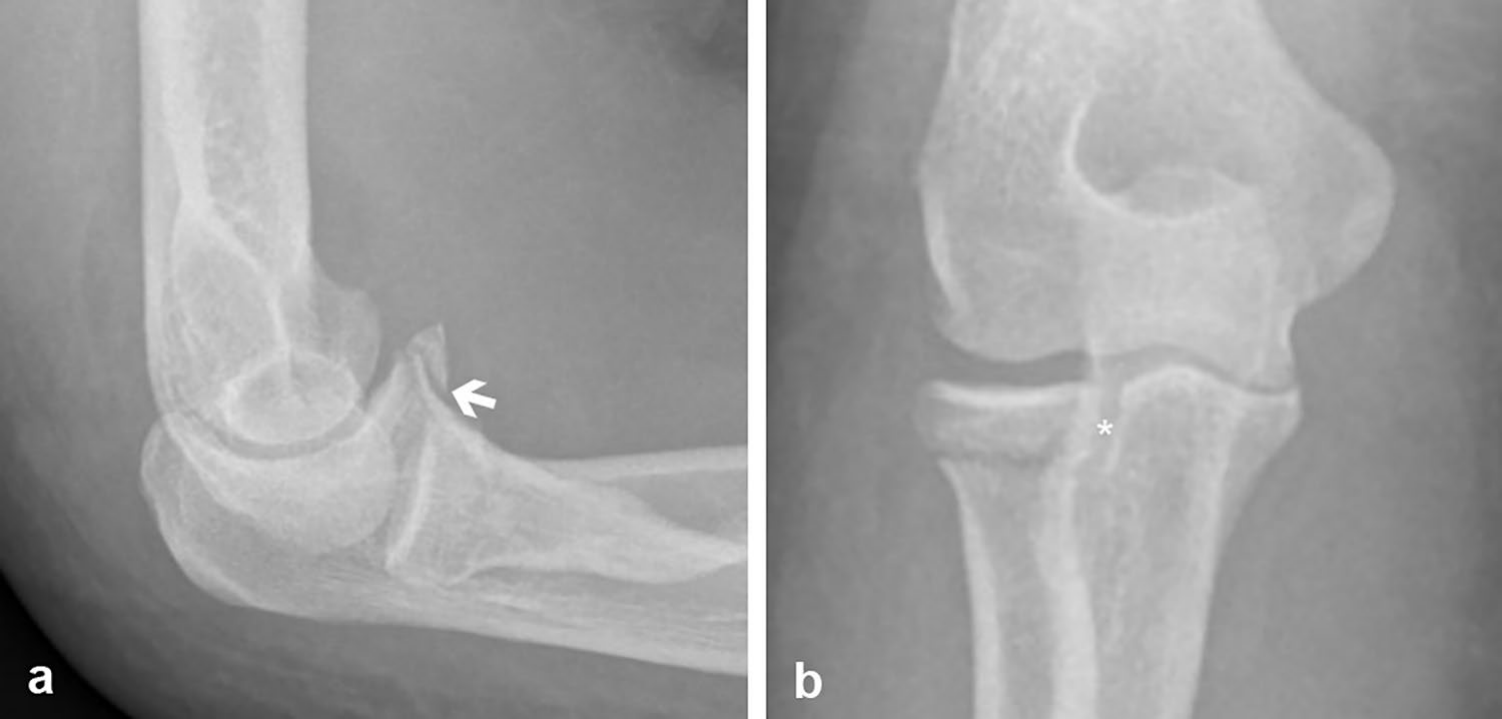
a, b Lateral (a) and anterior–posterior (b) radiographs from the same patient as in Fig. 1 a, b. Based on the radiographs, the fracture of the coronoid process (white arrow) may be classified as Regan and Morrey Type 1 or Type 2. The articular involvement of the radioulnar joint (white star) is not apparent from the radiographs alone, but requires a CT scan (Fig. 1 a, b)
